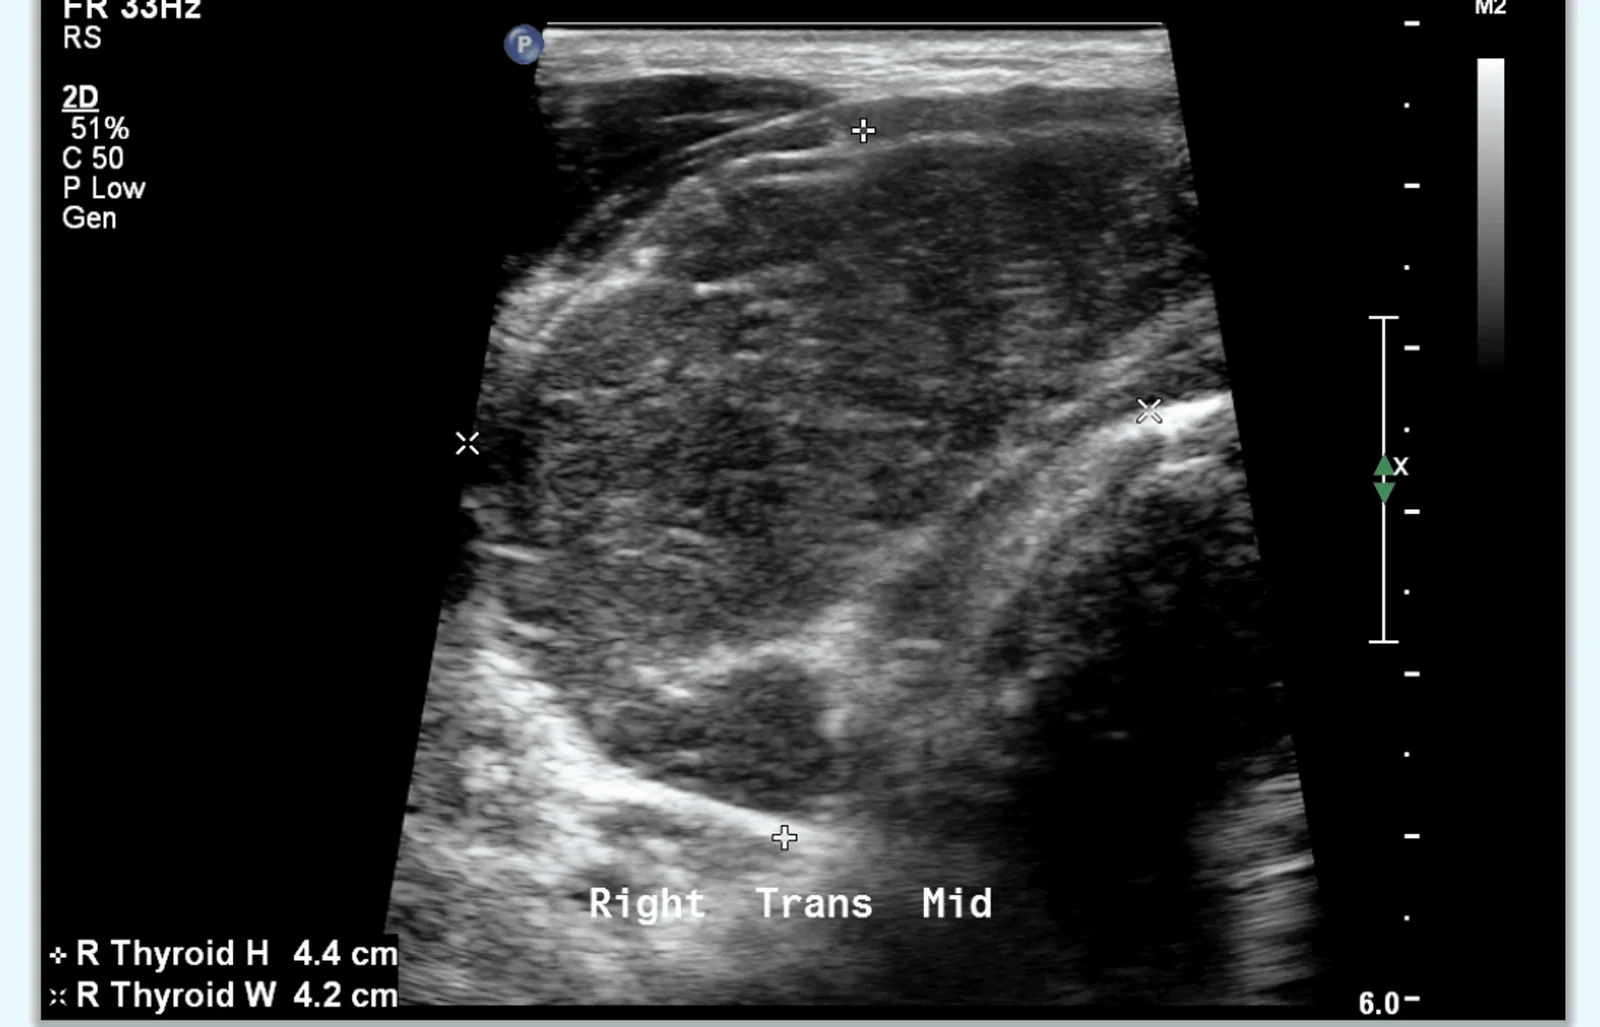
Transverse ultrasound view of the right lobe of the thyroid, measuring 4.4 x 4.2 cm.
